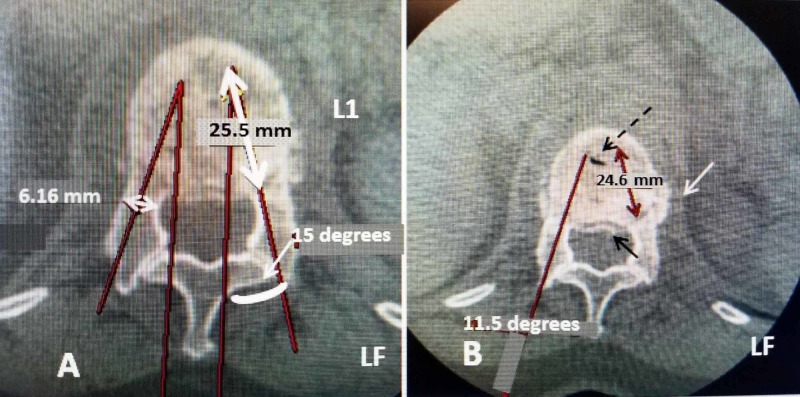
Measuring the fractured vertebral body to determine pedicle diameter and length to size correct implant to be inserted A: Axial CT scan of L1 fracture. The red line through the pedicle shows the angle (15 degrees) and position for placement of the implant. The 25.5-mm measurement is the maximum length of the implant (double-headed white arrow) on left (LF). The pedicle diameter 6.16 mm is also marked (small double-headed arrow on right over pedicle) to get the width of the closed implant that can be inserted. The final implant size is determined by both pedicle diameter (between the side cortex) and length to properly center the implants under the fractured endplates. In this case, a 5.8 x 20-mm implant can be used. B: Axial slice showing the angle (11.5 degrees) of insertion of the implant (solid red line). The fracture starts from the base of the L1 pedicle on the left side (LF) (solid white arrow). Minimal posterior displacement of the fracture into the spinal canal is seen (small solid black arrow). The length of the fractured endplate of 24.6 mm is measured (double-headed red arrow) which determines the maximum size implant that can be centered under the fracture. The slight lateral displacement of the fracture on the left can be seen (solid white arrow). A vertebral cleft is also identified in L1 in the anterior part of the fracture (dashed black arrow). The two SpineJack implants will be positioned anteriorly on each side of the cleft and cement will fill the cleft defect between the two implants.
